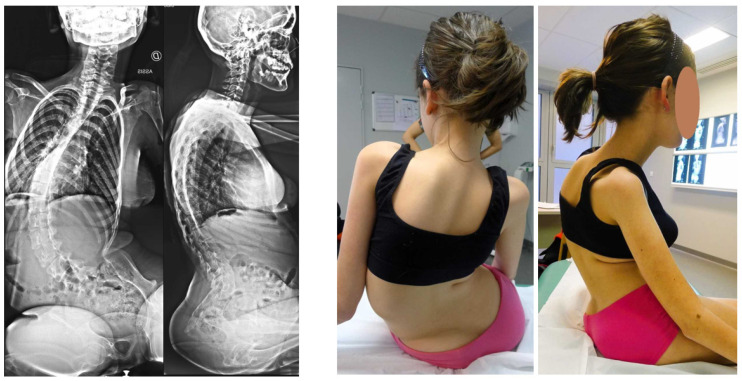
Preoperative X-rays and clinical pictures of a SMA2 patient.
